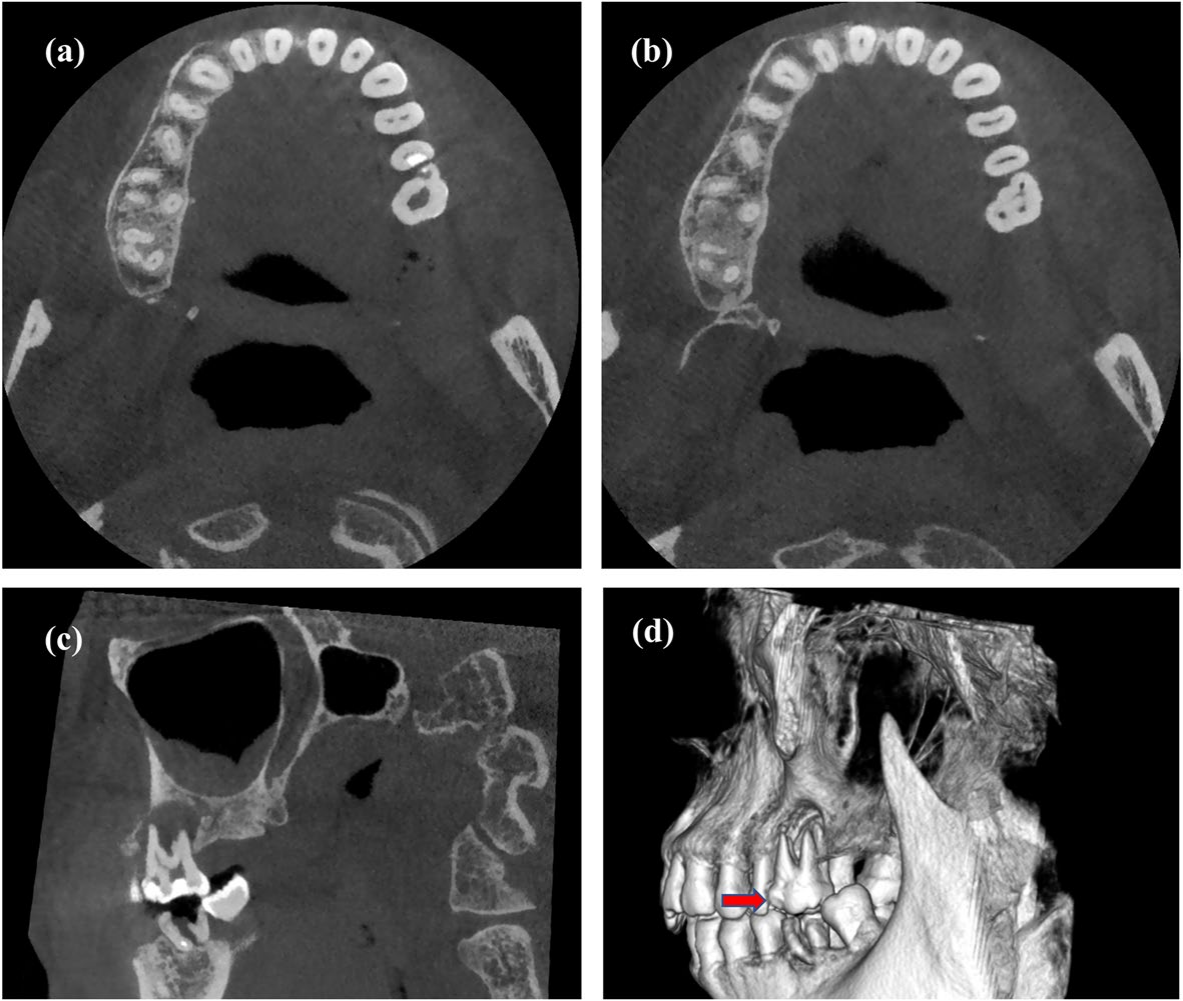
Cone-beam computed tomography images. a, b Cross section of plane of protostylid in tooth 26; (c) Vertebral plane of protostylid in tooth 26; (d) 3D reconstruction of protostylid in tooth 26. Red solid arrows indicate protostylid
